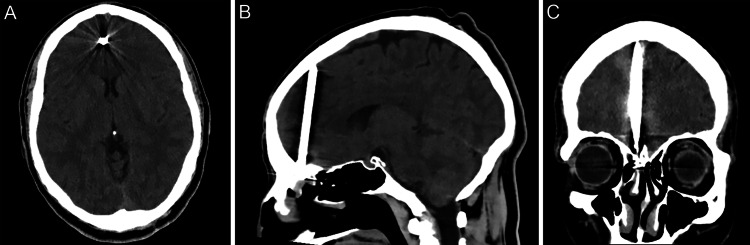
Preoperative computed tomography showing a transcribriform penetrating head injury caused by a nail gun. (A) Axial, (B) sagittal, and (C) coronal computed tomography showing an 8.3 × 0.5-cm nail penetrating the patient’s right cribriform plate and fovea ethmoidalis, projecting along the right mesial frontal lobe, and abutting the frontal inner table.* Used with permission from Barrow Neurological Institute, Phoenix, Arizona.*
